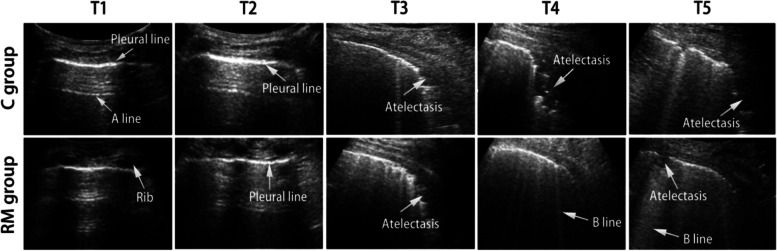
Lung ultrasound images of one representative patient per group
